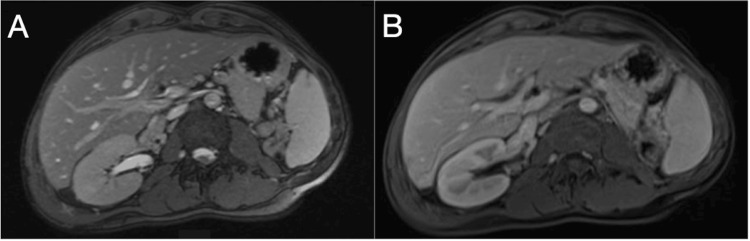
Post-transplantation MRI (A-B) Post-transplant graft with a normal-appearing parenchyma.
